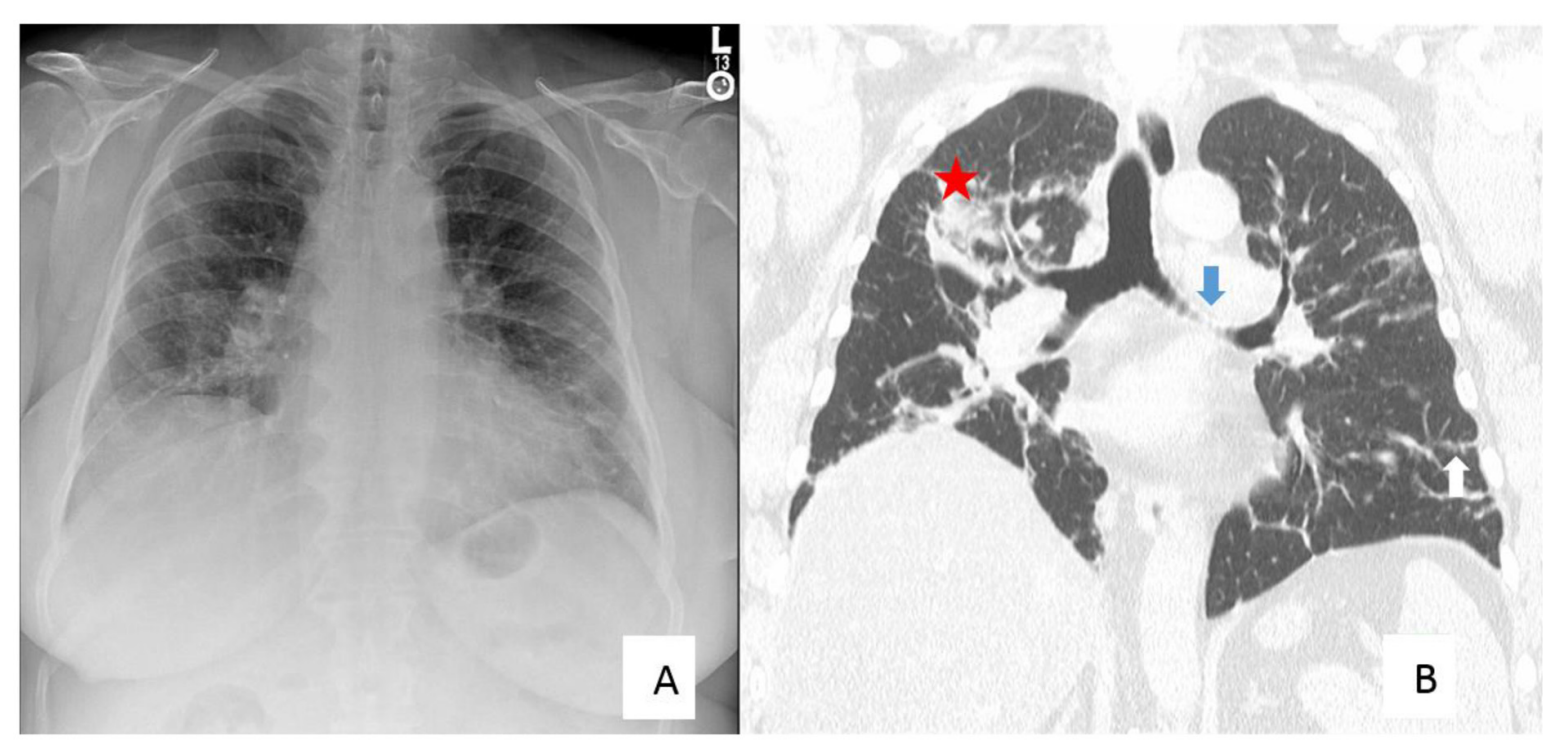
A chest X-ray and high-resolution computed tomography from the same patient are depicted. (
